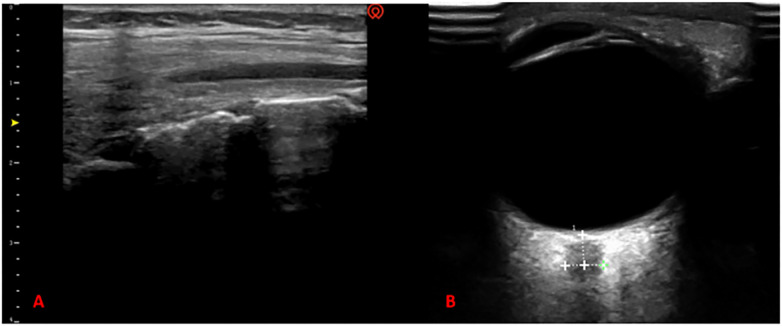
(A) the caudal block was administed with visual confirming by ultrasonography. (B) Measurement of optic nerve sheath diameter (ONSD) by ultrasonography. 1 vertical axis, 3 mm posterior to the optic nerve head; 2 horizontal axis, the width of ONSD.
